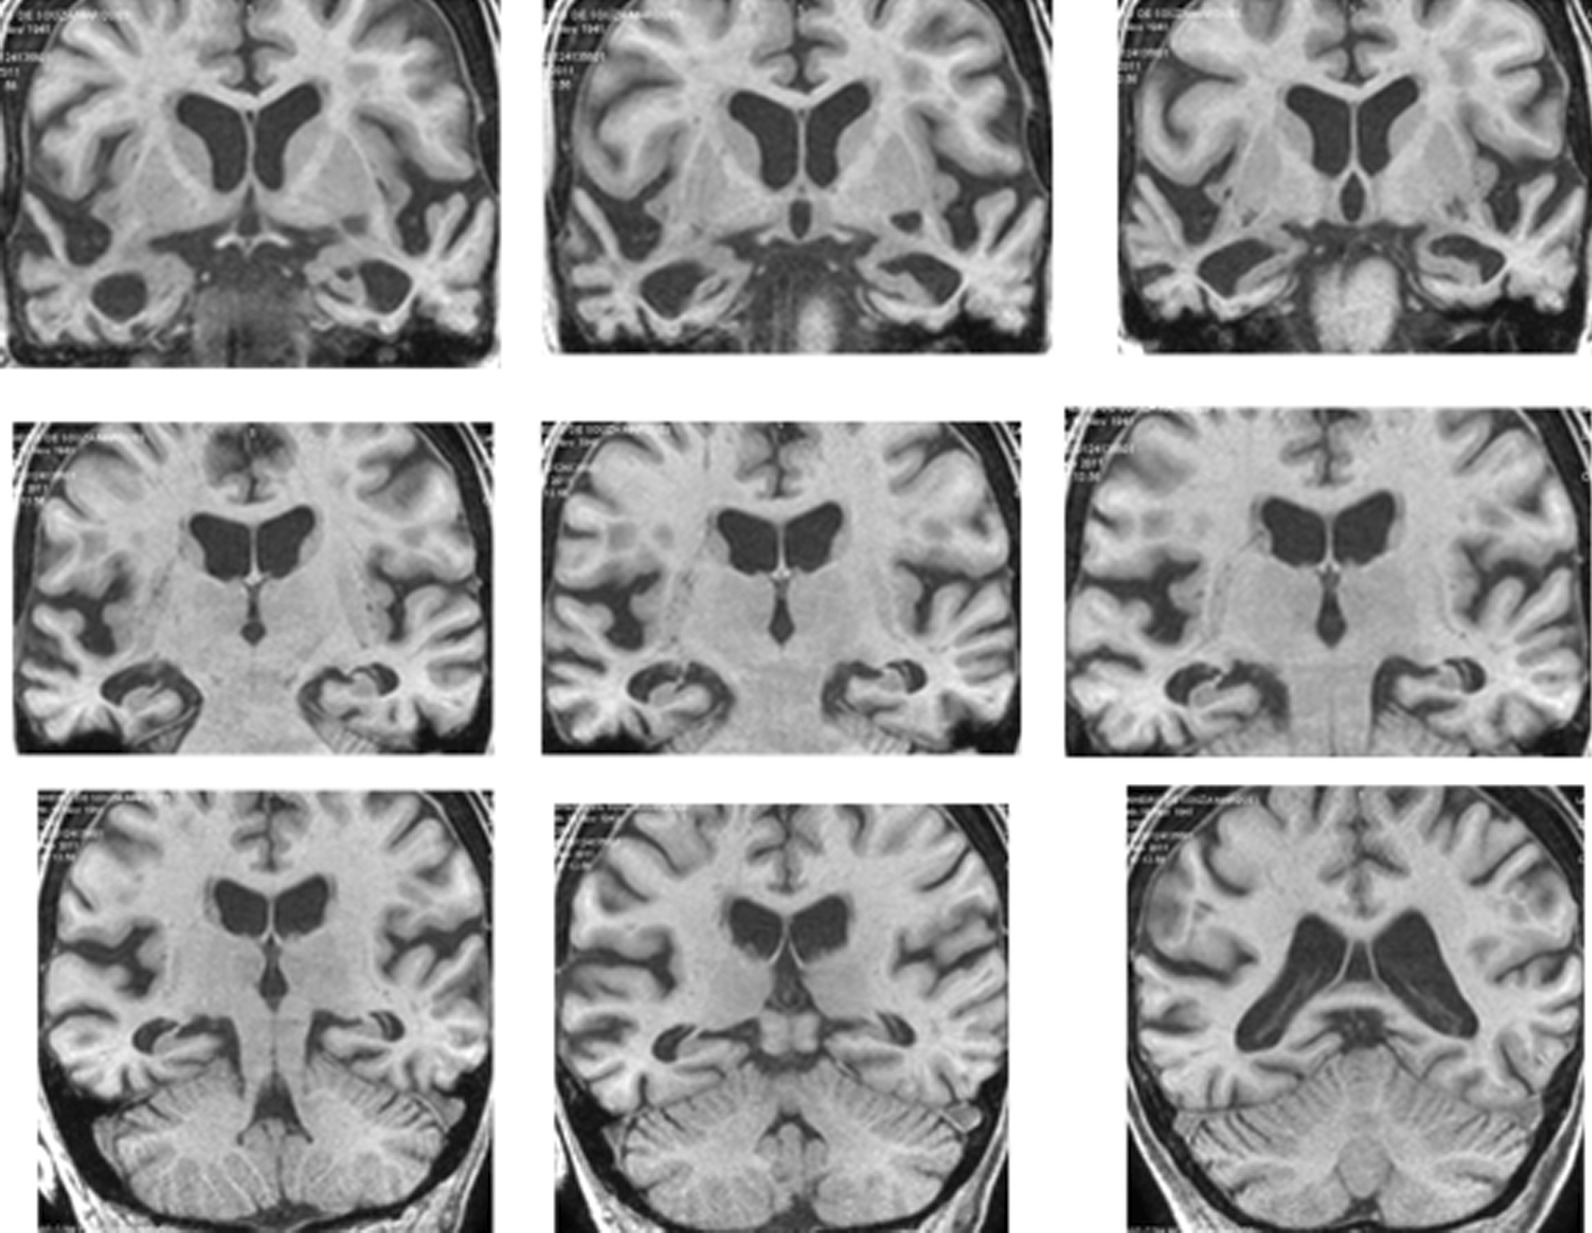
T1-weighted MRI coronal slices through the anterior (upper row), middle (middle row), and posterior (bottom row) third of the cerebral hemispheres. Atrophy is most marked in the temporal lobes, more so on the right, as indicated by the wider Sylvian fissure and temporal horn on this side. The lateral ventricles are also symmetrically enlarged
